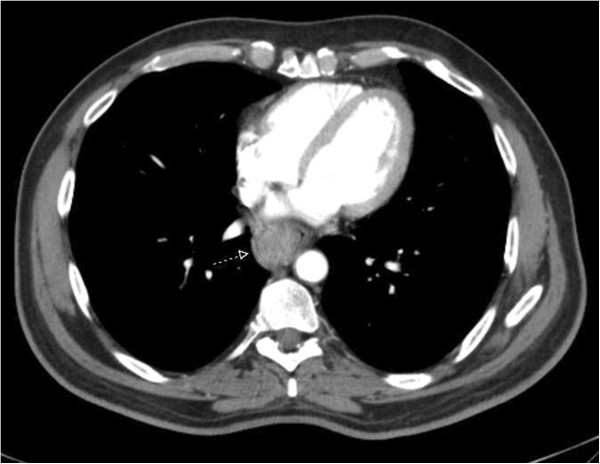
CT scan shows a bulky tumor in the lower third of the esophagus (arrow).
